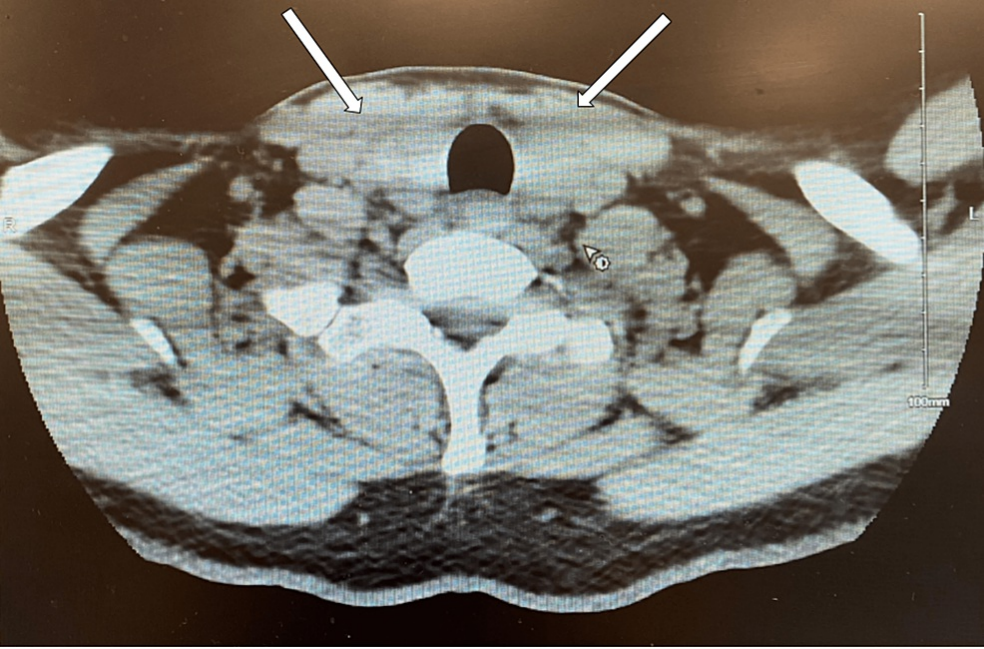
CT neck showing heterogeneous and prominent thyroid nodules.
